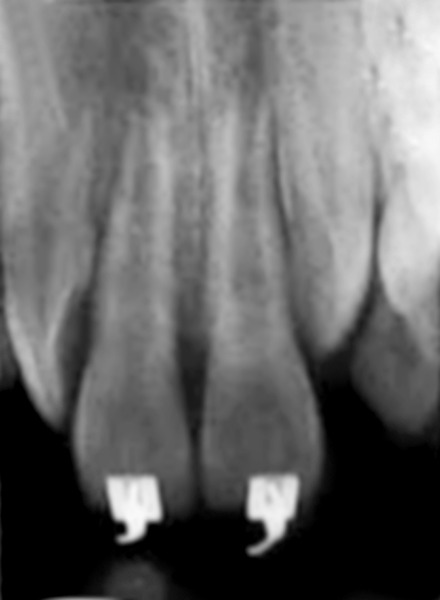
Periapical radiograph showing no bone resorption with proper alignment
